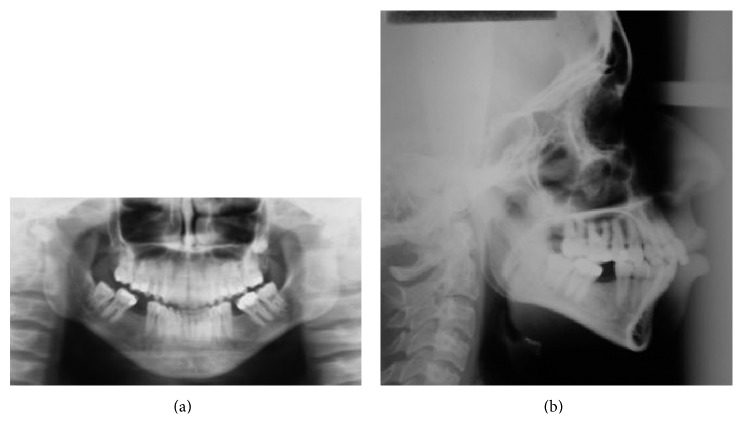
Pretreatment panoramic (a) and lateral (b) radiographs at 19 years, 0 month.
